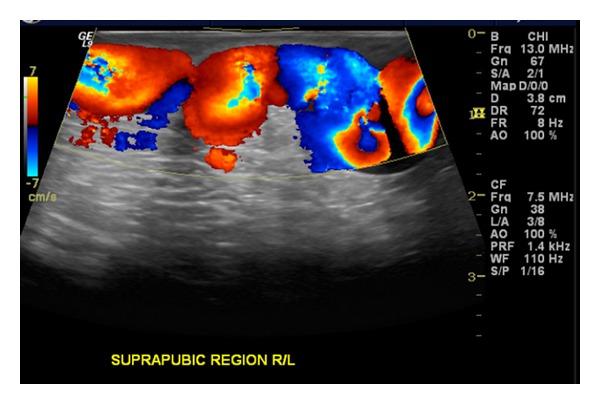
Duplex ultrasound of suprapubic venous plexus. Note the tortuosity in the vessel and the continuous flow beneath the ultrasound probe.
